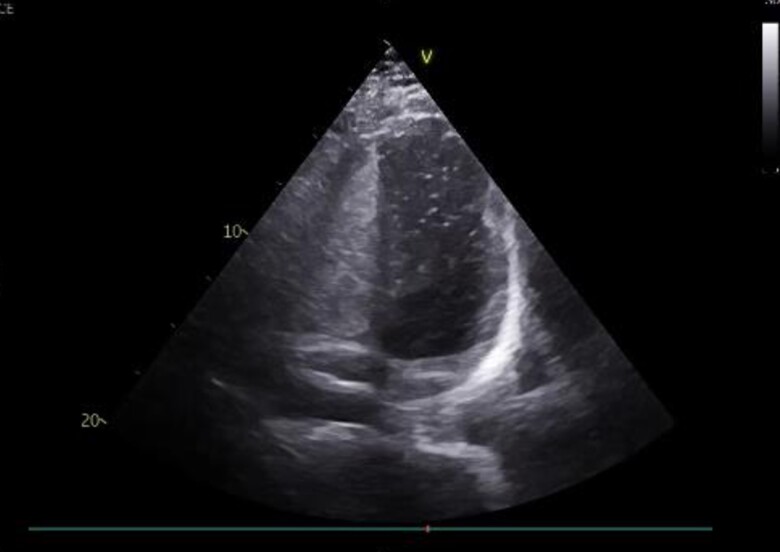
A bubble study transthoracic echocardiogram showing right-to-left shunt.
